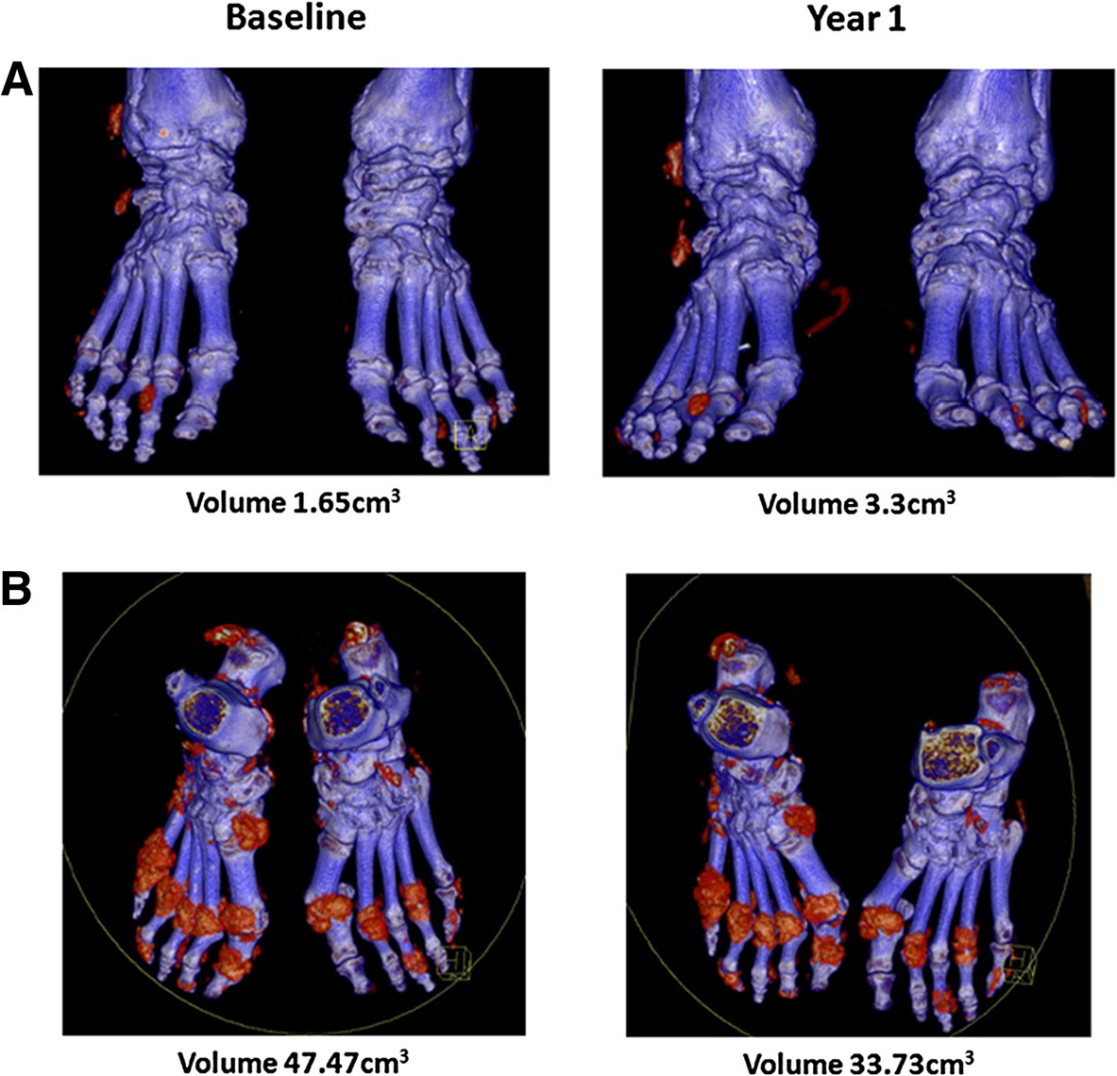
Examples showing lack of association between changes in serum urate concentration and changes in dual-energy computed tomography urate volume. Paired dual-energy computed tomography (DECT) scans from two patients with gout with hyperuricaemia throughout the study period. (A) Paired scans from a patient with 0.49 mmol/L serum urate concentration at baseline visit, and 0.57 mmol/L at year 1 visit. DECT urate volume increased from 1.65cm^3^ to 3.3 cm^3^. (B) Paired scans from a patient with a 0.55 mmol/L serum urate concentration at baseline and 0.56 mmol/L at year 1. DECT urate volume decreased from 47.47 cm^3^ to 33.73 cm^3^.
